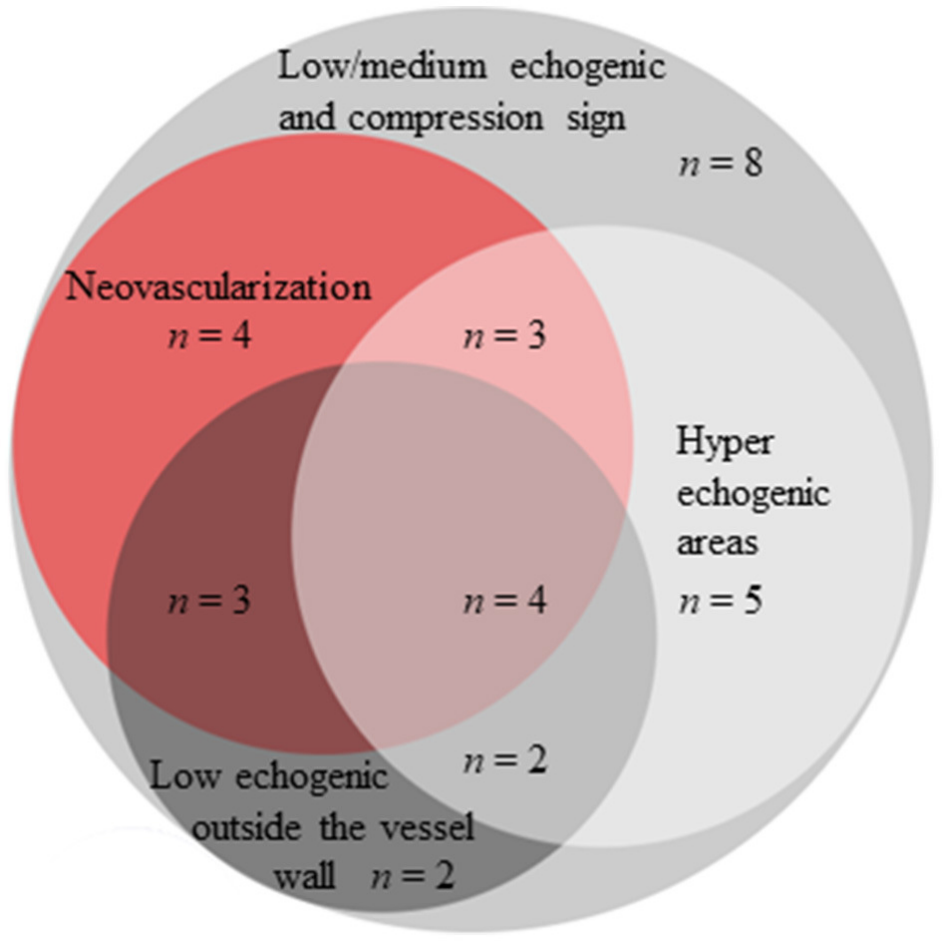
Morphological findings in the temporal arteries of patients with GCA using high-frequency ultrasound and SMI.
